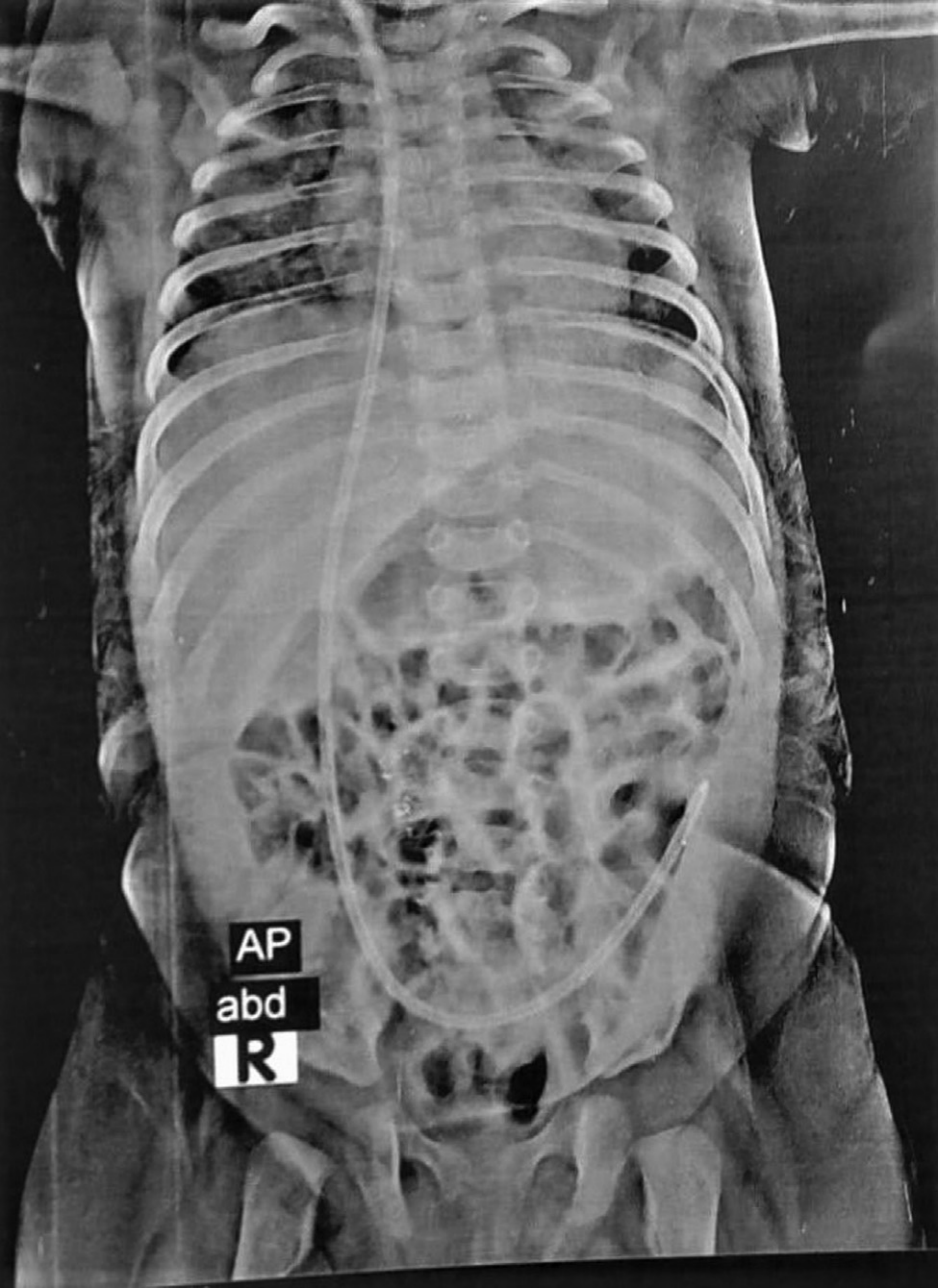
Postoperative plain X-ray of abdomen and pelvis showing the distal tube properly replaced.
